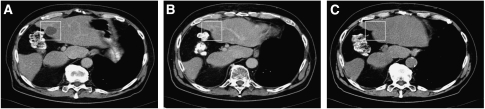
CT scans of a representative liver metastasis from patient B. (A) Liver metastasis at baseline just before initiating sunitinib. (B) Liver metastases after 6 months sunitinib. (C) Liver metastases after 18 months sunitinib.
